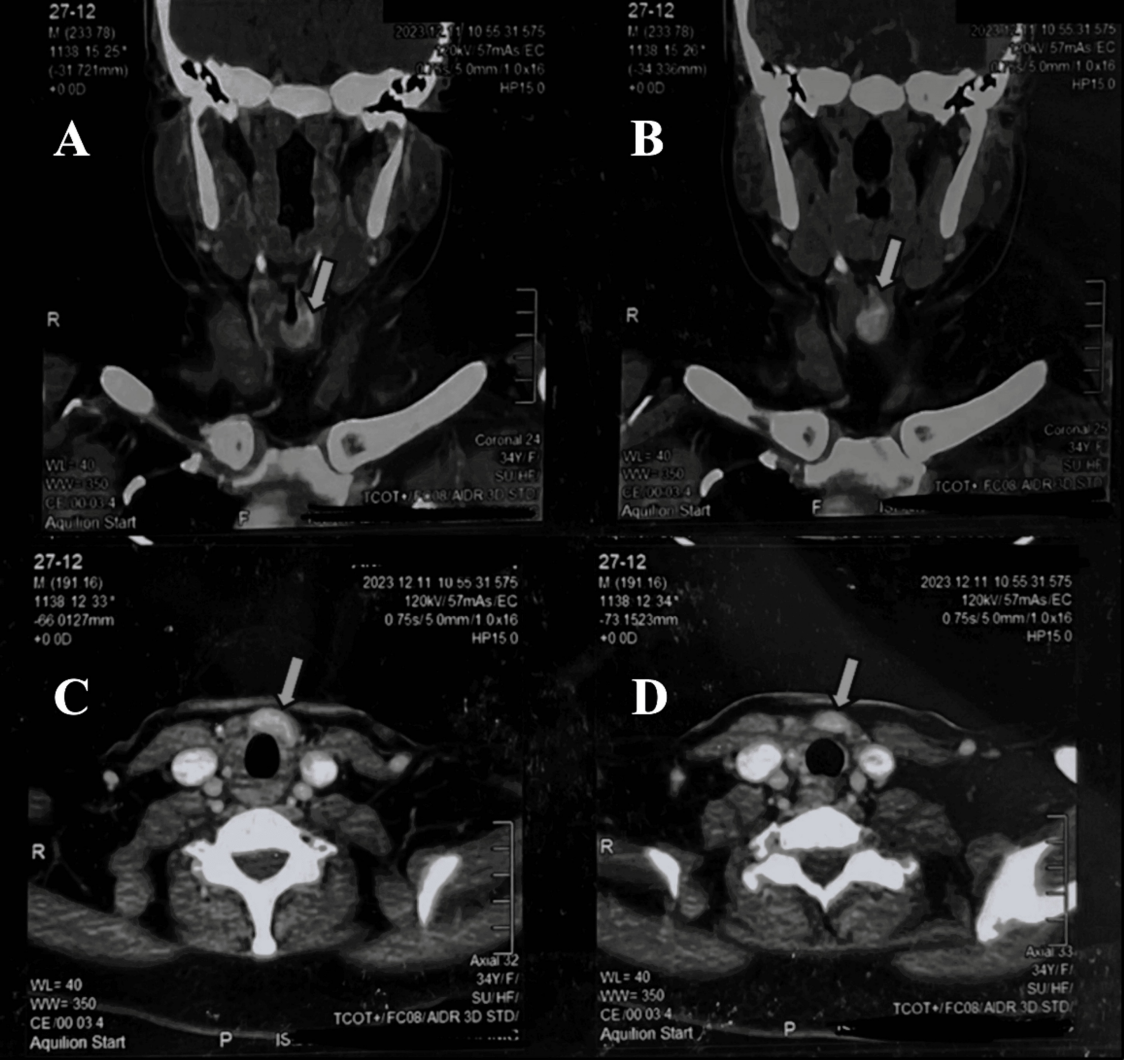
Contrast-enhanced computed tomography neck showing thyroid remnant at the level of isthmus (post-total thyroidectomy) A and B: coronal view showing thyroid remnant as shown by the arrow; C and D: axial view showing thyroid remnant as shown by arrow
